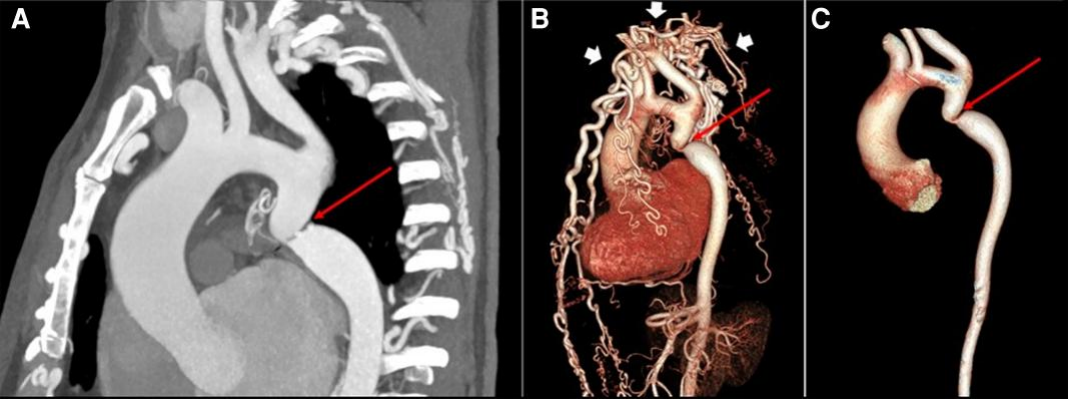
(*A*) Sagittal oblique reformatted maximum intensity projection image from cardiac computed tomography angiography demonstrates a focal severe coarctation (arrow) of the aorta with multiple collateral arteries. (*B* and *C*) Three-dimensional volume-rendered image in left lateral view shows prominent collateral arteries (white arrowheads) due to severe coarctation of the aorta (arrow).
